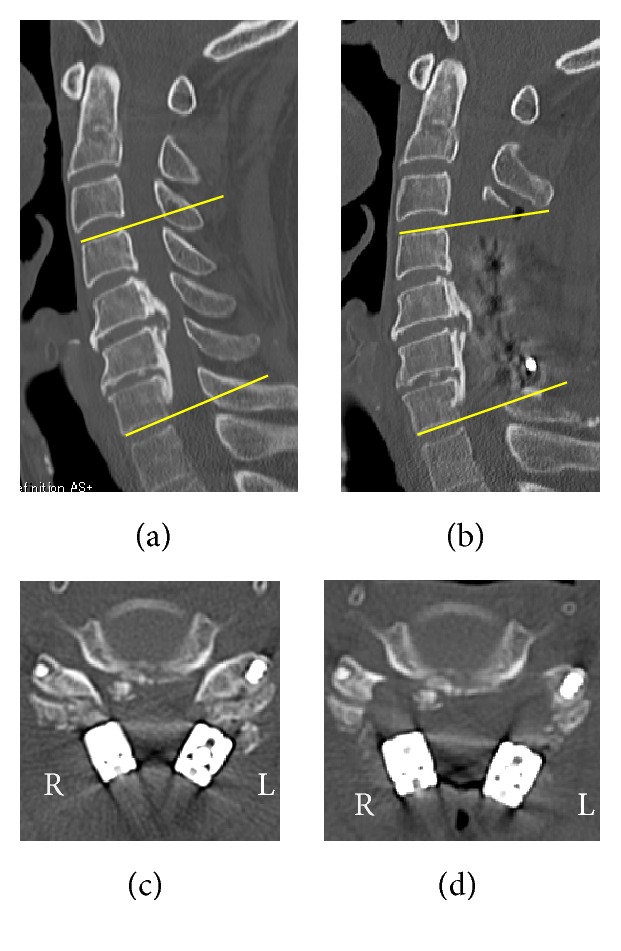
Pre- (a) and postoperative ((b) and (c)) and postadditional foraminotomy (d) computed tomography (CT). (a) and (b): lordosis angle at the operated segment (C4–7) increased by 5 degrees compared to the preoperative angle. (c): there were no changes in anteroposterior diameters of the bilateral C4/5 foramen and no malposition of the screws on postoperative CT. (d): Additional bilateral foraminotomy at C4/5 was performed.
